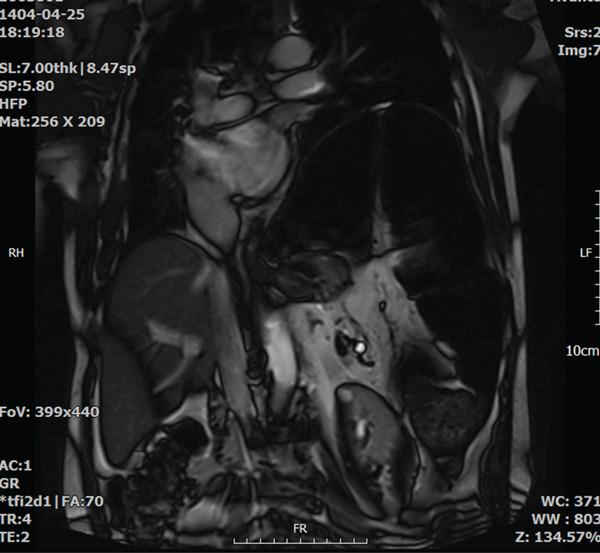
Coronal MRI scan. Demonstrating congenital diaphragmatic eventration with herniation of dilated bowel loops into the thoracic cavity.
